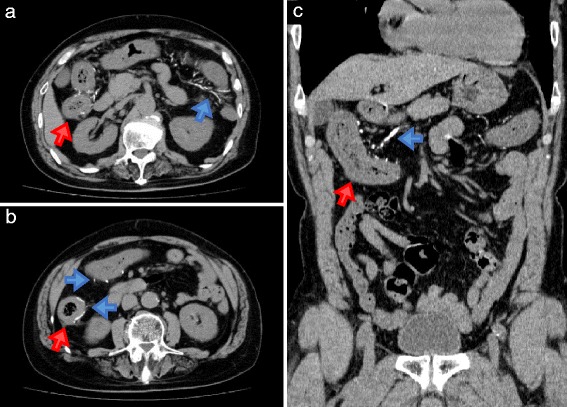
Typical findings of CT scan in MP cases (No. 2 in Table 2). Typical calcification and wall thickening in the transverse colon (a) and the ascending colon (b). Linear calcification in the transverse colon in coronal section (c). The blue colored arrows indicate the typical calcification. The red colored arrows indicate the thickening in the colon. CT, computed tomography; MP, mesenteric phlebosclerosis
